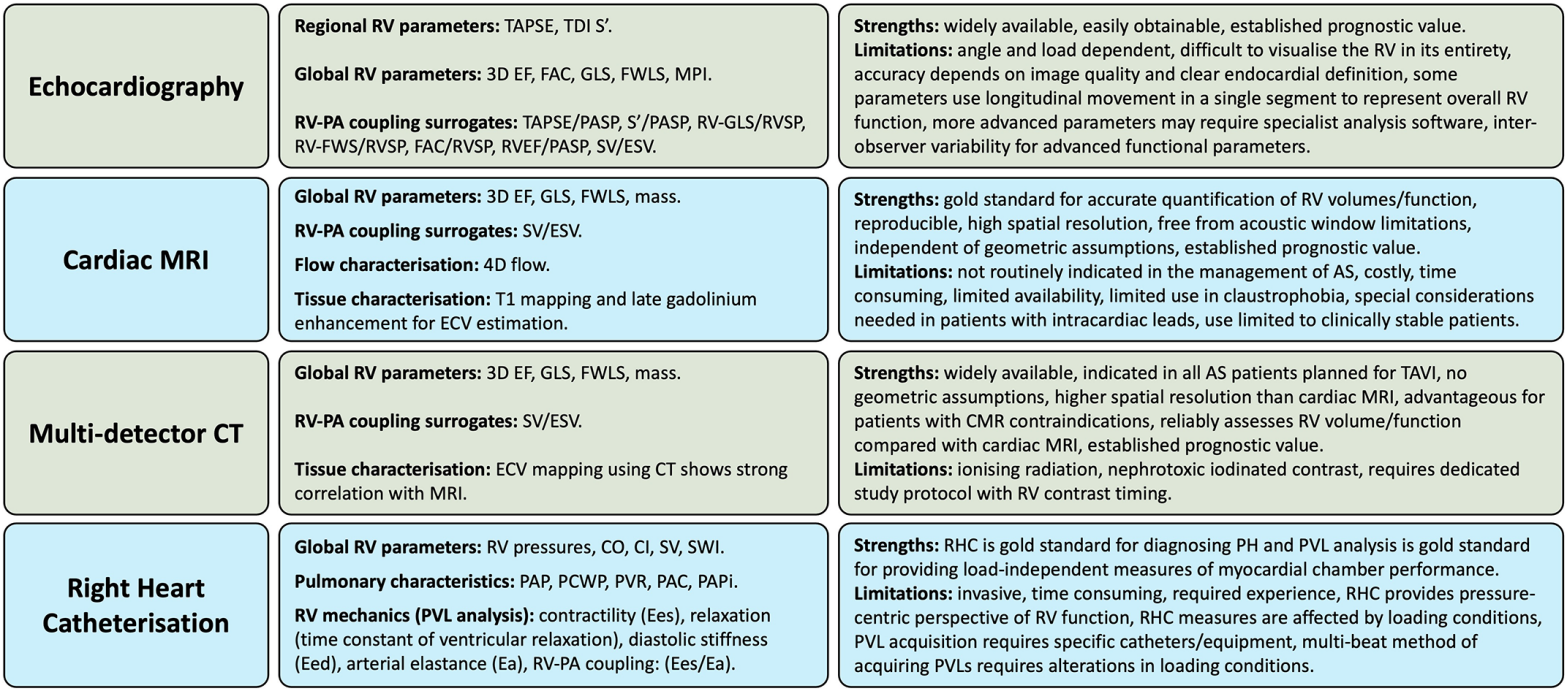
Multi-modality approach to assessing right ventricular function in aortic stenosis. AS, aortic stenosis; CO, cardiac output; CI, cardiac index; CT, computed tomography; Ea, arterial elastance; Eed, end-diastolic elastance; Ees, end-systolic elastance; EF, ejection fraction; ESV, end-systolic volume; FAC, fractional area change; FWLS, free-wall longitudinal strain; GLS, global longitudinal strain; mPAP, mean pulmonary artery pressure; MPI, myocardial performance index; MRI, magnetic resonance imaging; RV, right ventricle, PA, pulmonary artery; PCWP, pulmonary capillary wedge pressure; PAC, pulmonary arterial compliance; PAPi, pulmonary arterial pulsatility index; PAP, pulmonary artery pressure; PASP, pulmonary artery systolic pressure; PH, pulmonary hypertension; PVL, pressure-volume loop; PVR, pulmonary vascular resistance; RHC, right heart catheterisation; RVSP, right ventricular systolic pressure; SV, stroke volume; SWI, stroke work index; TAPSE, tricuspid annular plane systolic excursion; TDI, tissue doppler imaging; 3D, 3-dimentional.
